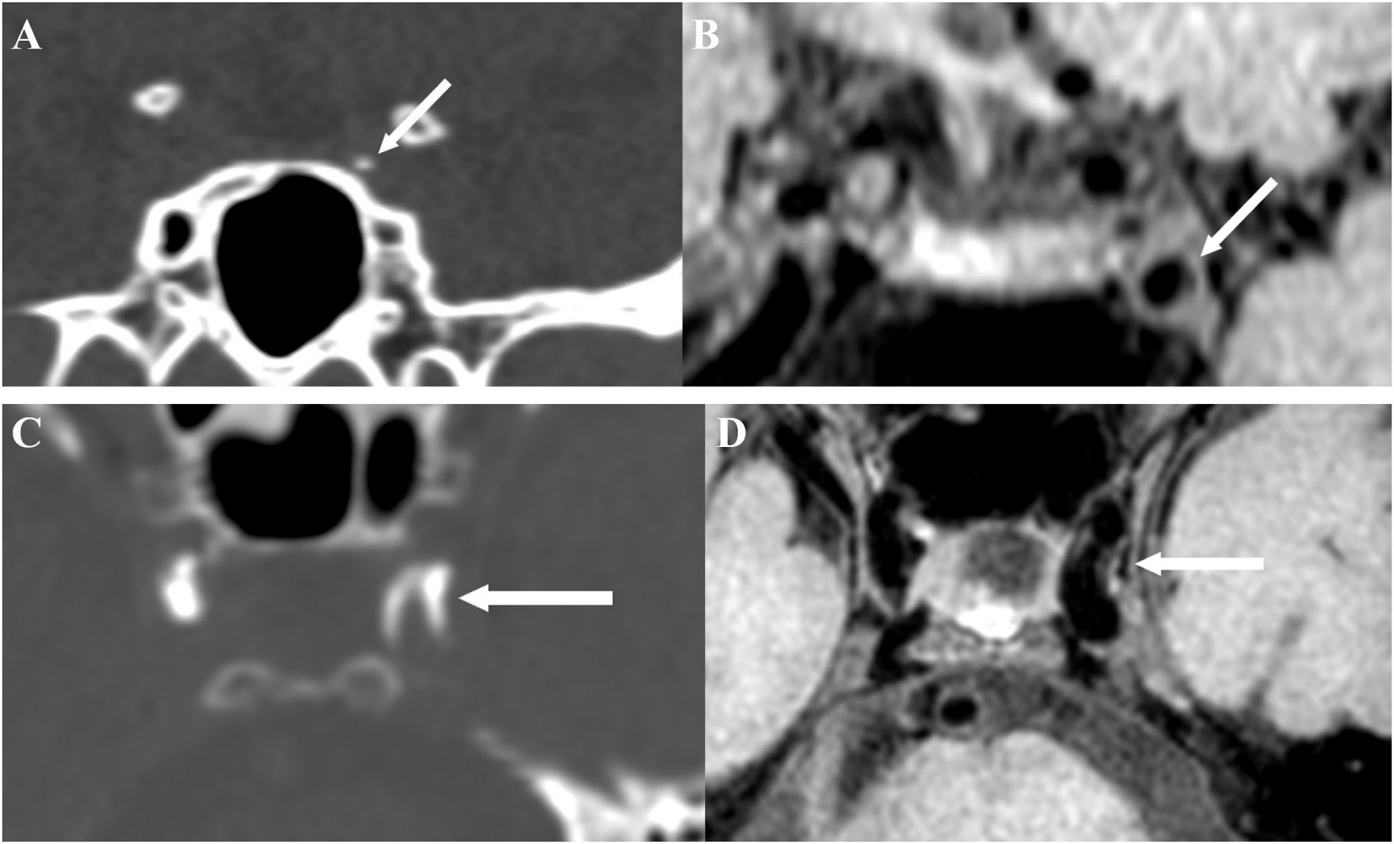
Intimal calcification detected by brain CT (A) and the corresponding site on vessel-wall MRI (vwMRI) (B). Medial calcification detected by brain CT (C) and the corresponding site on vwMRI (D).
